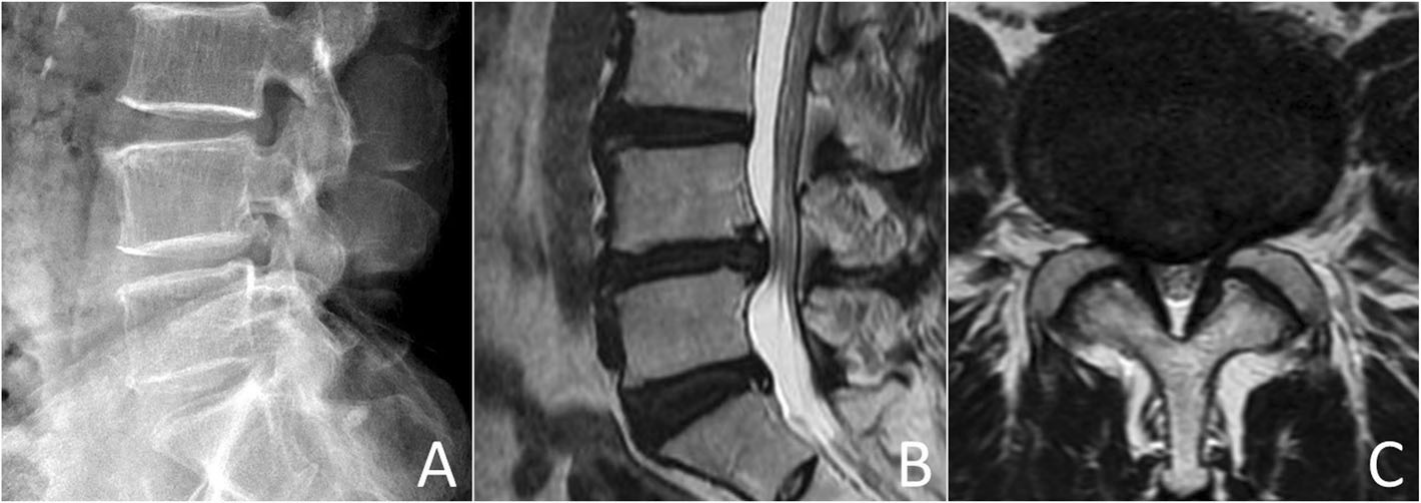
Pre-operative lateral radiograph (a), sagittal and axial (b and c) magnetic resonance images
